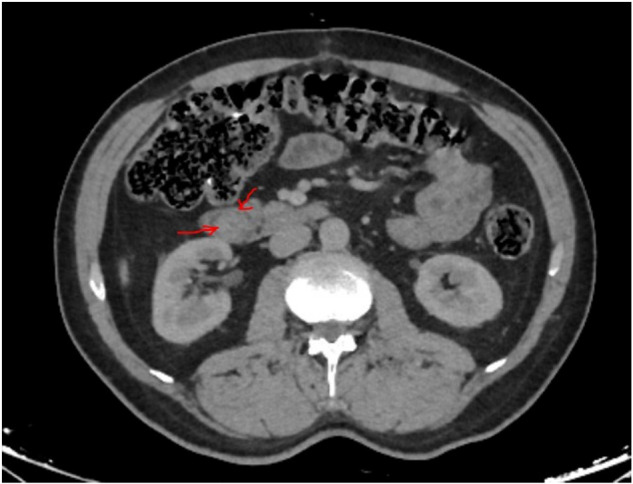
Contrast-enhanced CT demonstrating a small mass in the pancreatic head.
